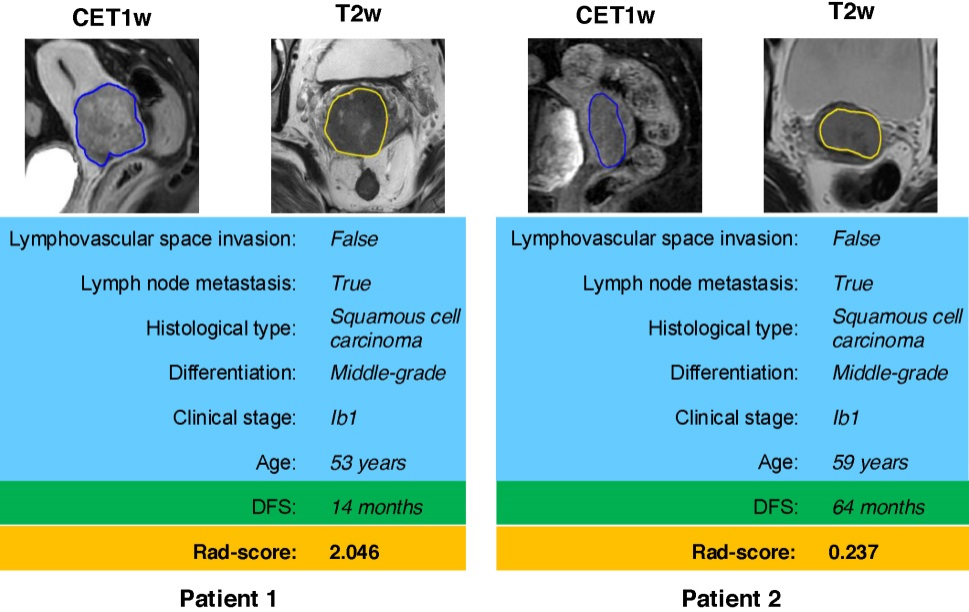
MR images of two patients with significantly different DFS time. Although patient 1 and patient 2 have similar clinicopathological characteristics, their Rad-score are different and discriminative. DFS: disease-free survival.
